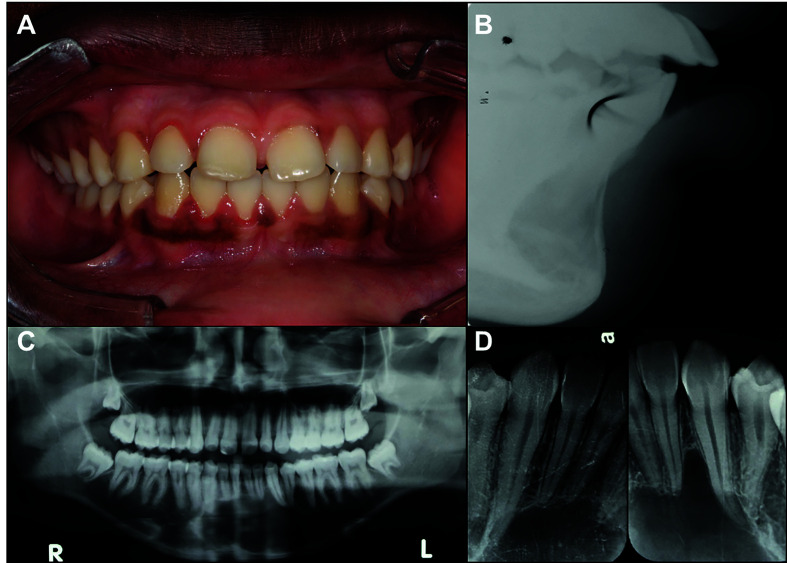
A: Intra-oral aspect of anterior SBC; B: Sagittal Radiographic aspect of the SBC. C: Panoramic radiograph showing SBC lesion in anterior portion of mandible; D: Periapical radiograph presenting scalloped margins between dental elements.
